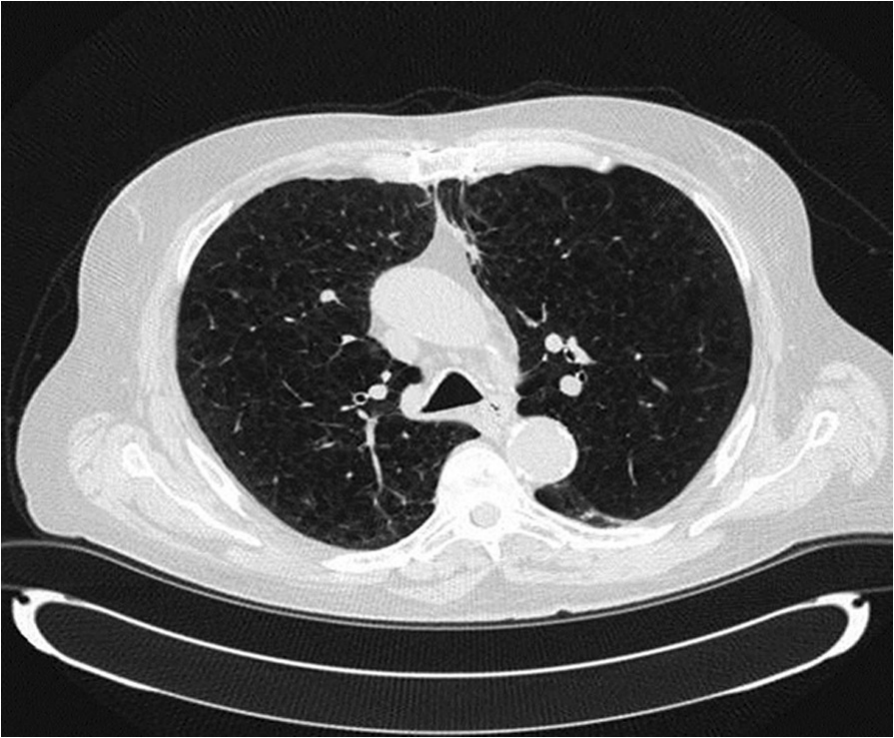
CT scan performed 2 months after SABR shows significant reduction in size of the treated lung lesion without evidence of radiation pneumonits.
